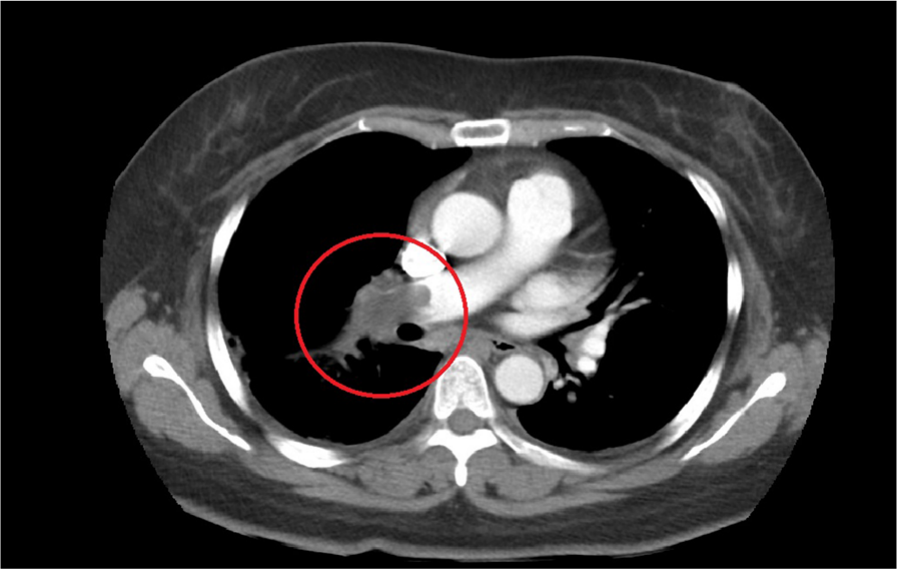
Thoracic contrast-enhanced computed tomography. Contrast-enhanced computed tomography scan revealing a massive pulmonary tumor embolism (circle) occluding the right pulmonary artery
